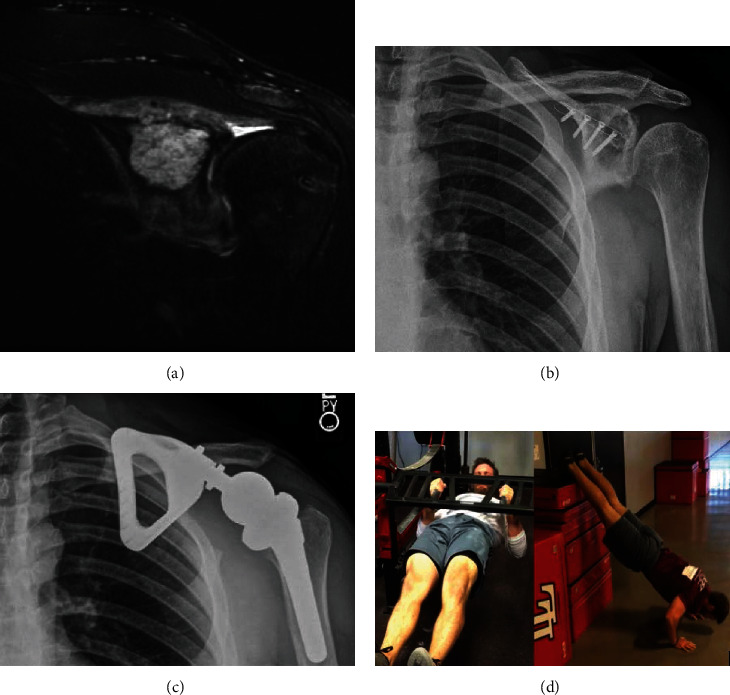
(a) MRI demonstrating malignant lesion of the scapula involving the subglenoid region of the shoulder. (b) Shoulder radiograph after index surgery which involved intralesional curettage, cryosurgery, grafting, and prophylactic internal fixation of the left glenoid. (c) Three-year postoperative radiograph after shoulder reconstruction. (d) Patient performing cross-fit activities postoperatively.
